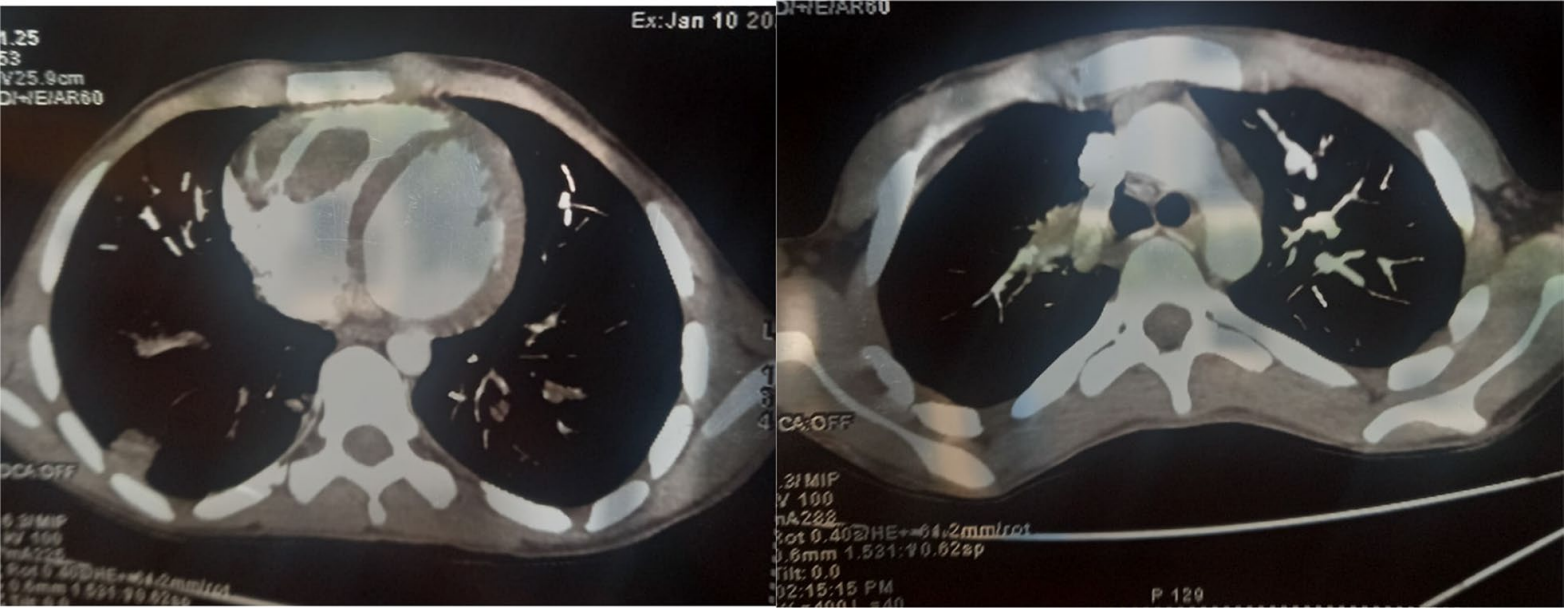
Thoracic Angio CT: bilateral pulmonary embolism associated with right ventricular mass
